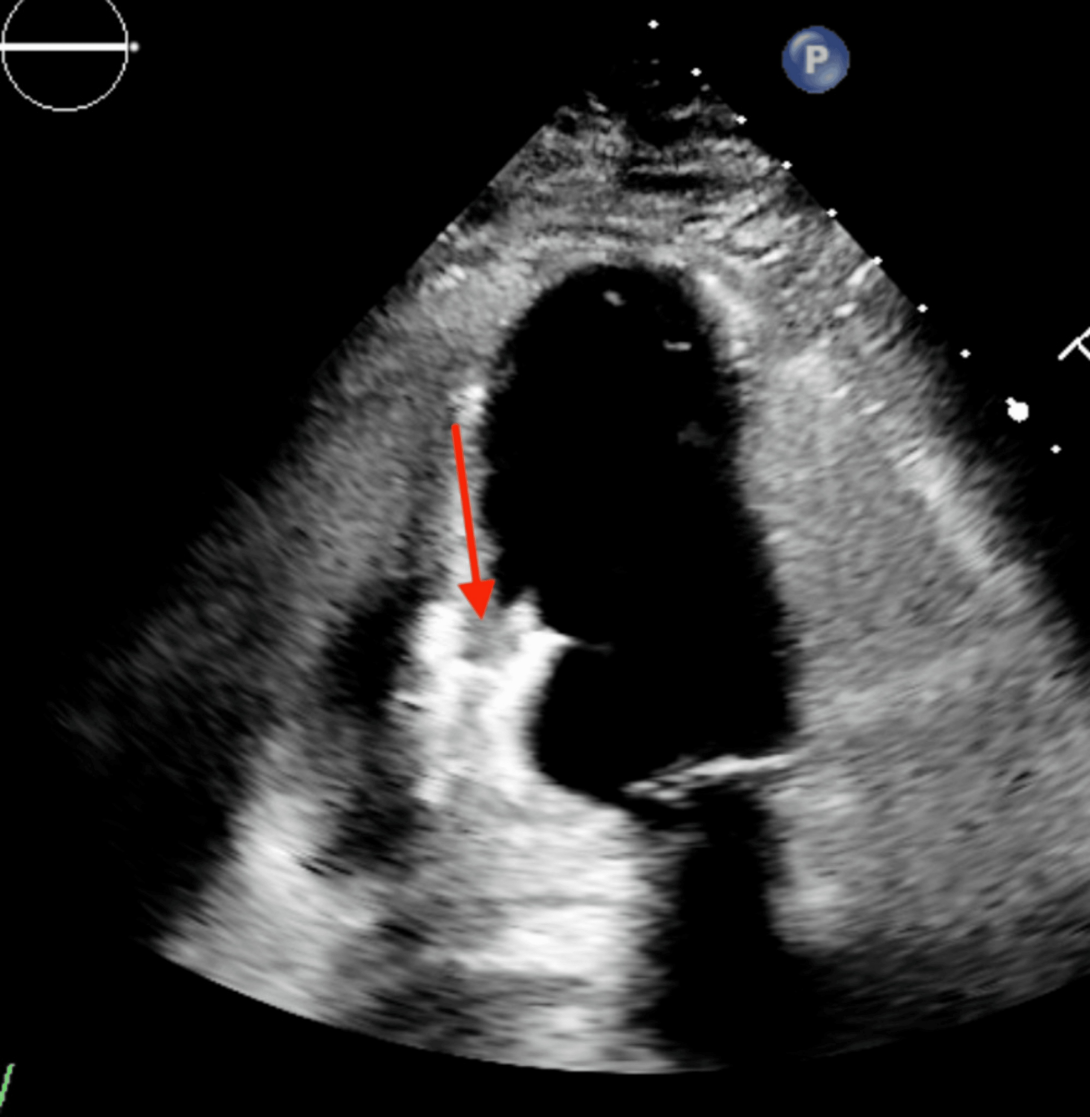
Echocardiogram after placement of the ventricular septal defect closure device implantation
